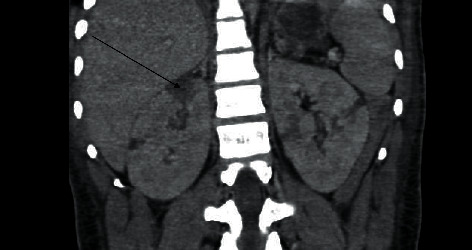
Computed tomography (CT) of the abdomen showing multiple hypodense wedge-shaped parenchymal defects involving the cortex and medulla consistent with renal infarcts (black arrow).
